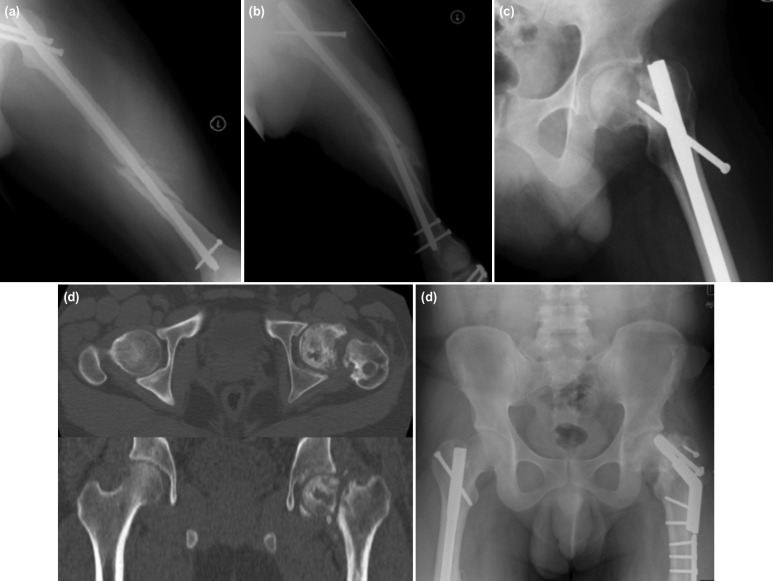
(a) Photos showing the initial reconstruction nail used to address the shaft and the femoral neck fractures in case no.3, (b) second traumatic event caused implant failure to the previously fixed femoral neck fracture, (c) radiological evidence of non-union over the femoral neck, (d) imaging from the CT scan showed more obvious radiological evidences of non-union and AVN of the femoral head, (e) radiograph four years after sliding compression screw and myo-osseous trochanteric flap showed that the neck already united, however there was head AVN and shortening of the femoral neck. Patient had 2cm shortening and MRC grade 3 hip abductor.
